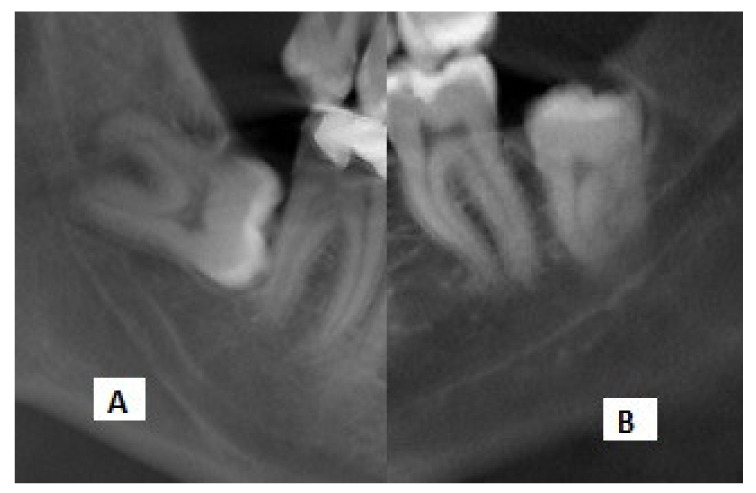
Cropped panoramic radiographs showing the (A) interference with the white line and (B) darkening of roots.
